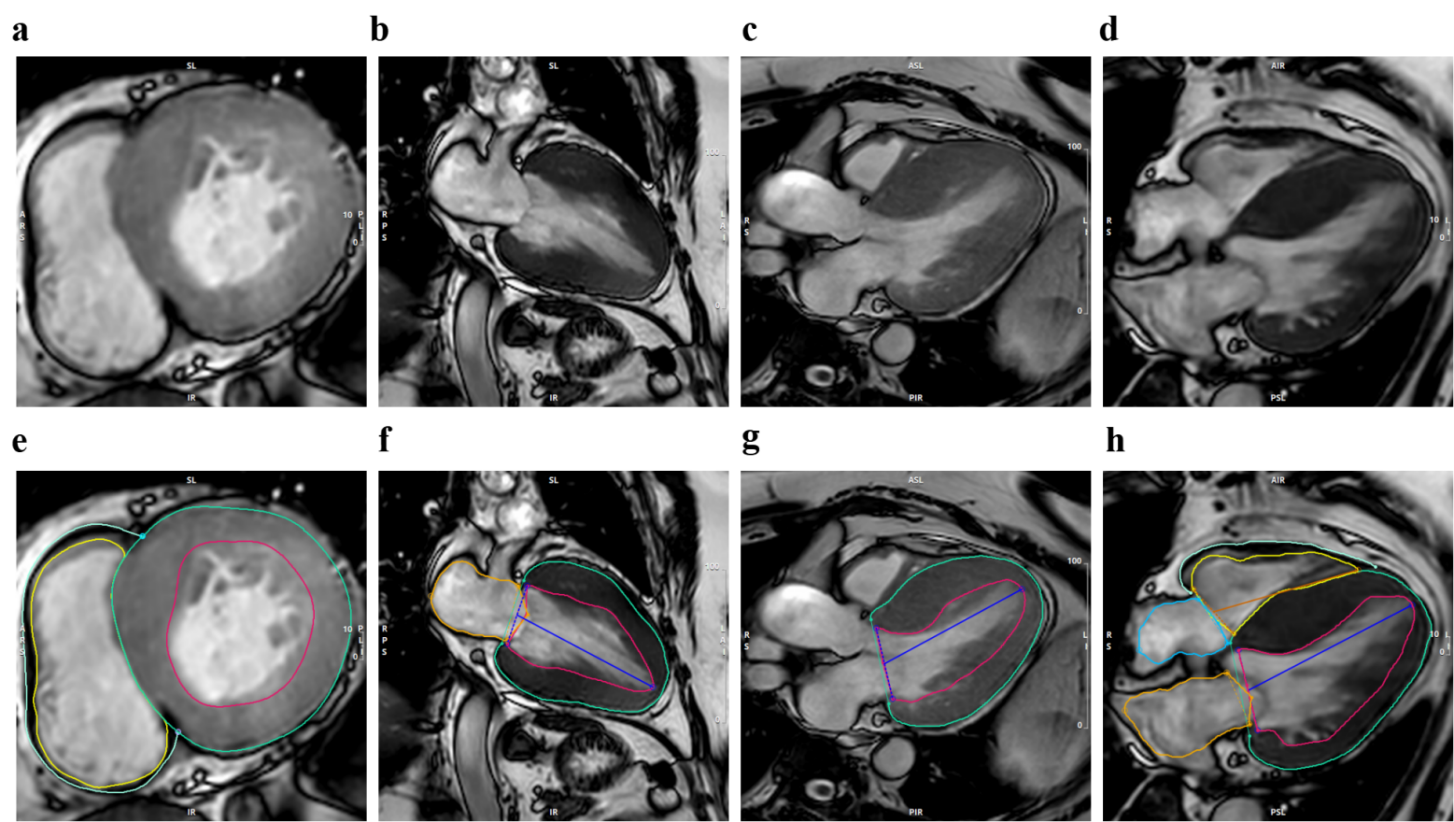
Typical cardiac magnetic resonance imaging (CMRI) presentation
